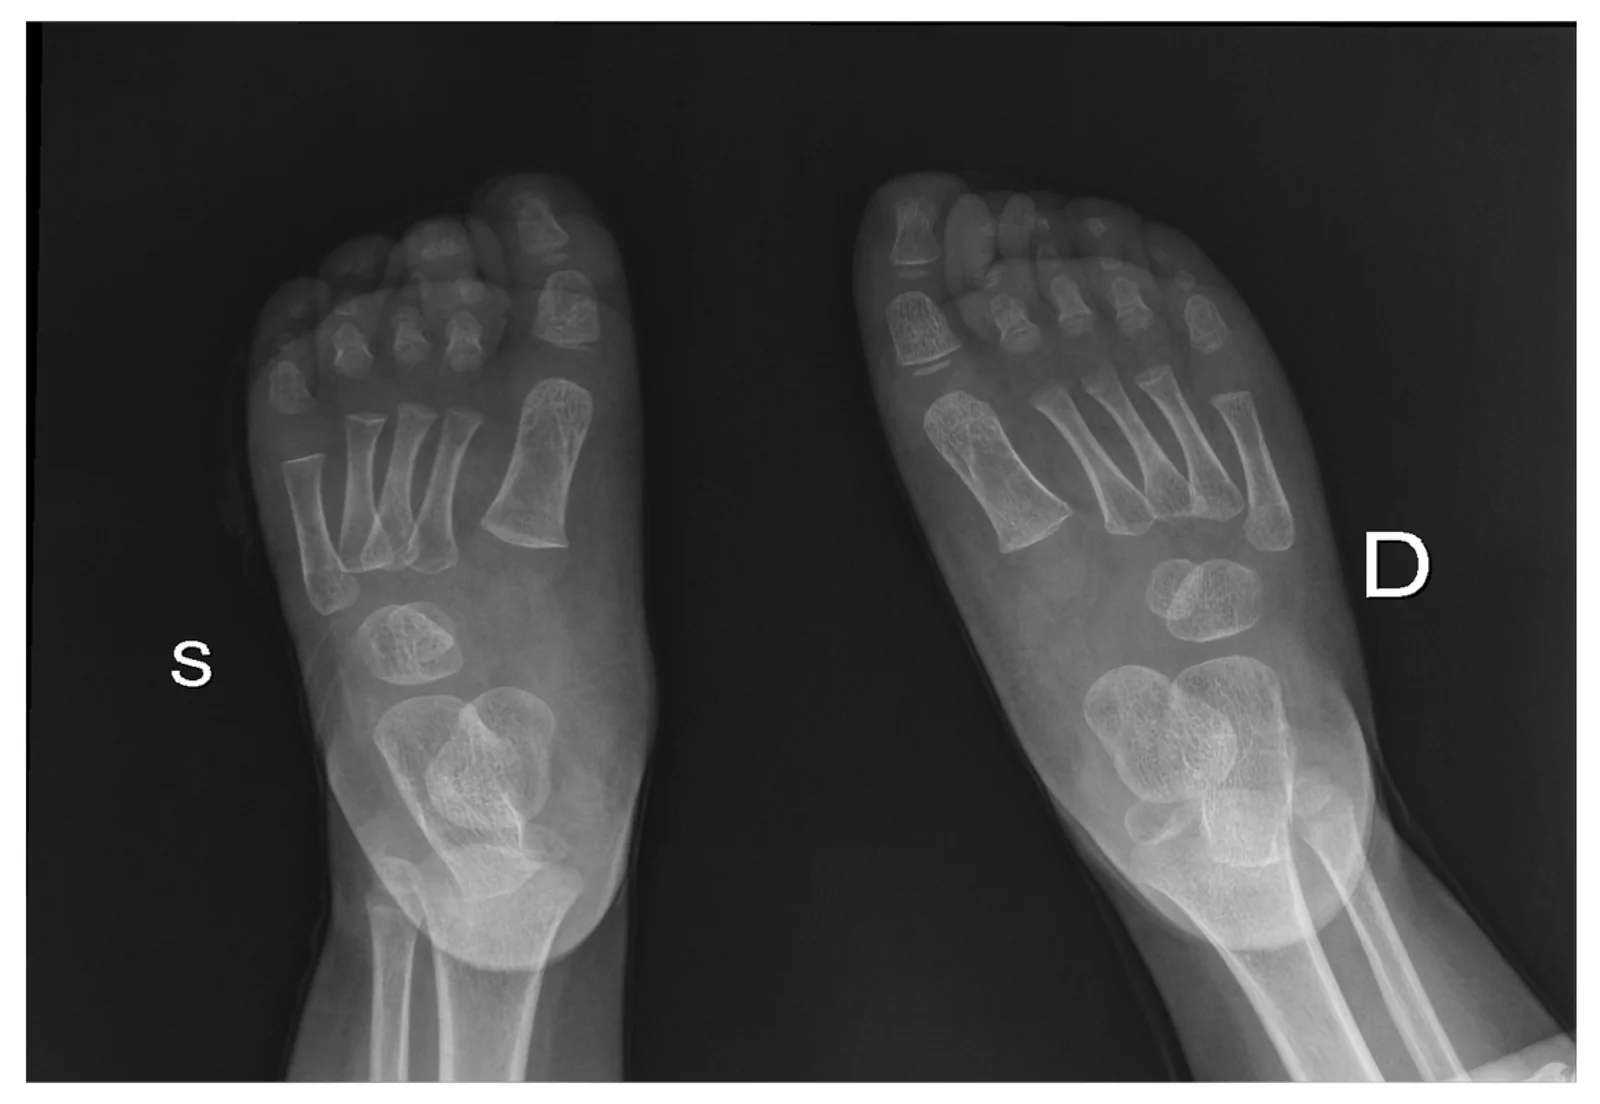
Foot radiographs demonstrating absence of the navicular ossification center.
